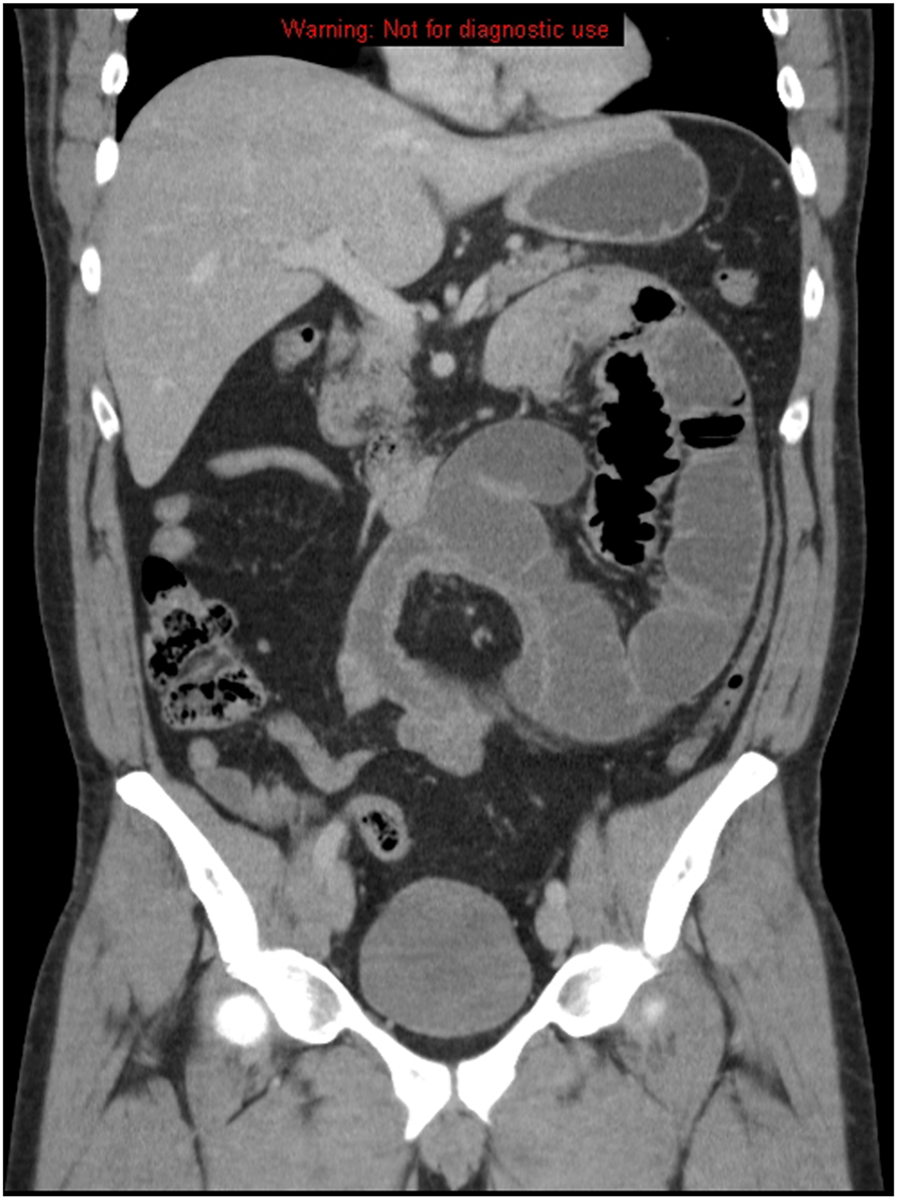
Axial enhanced CT demonstrates a cluster of dilated jejunal loops located in the Landzert´s fossa.
